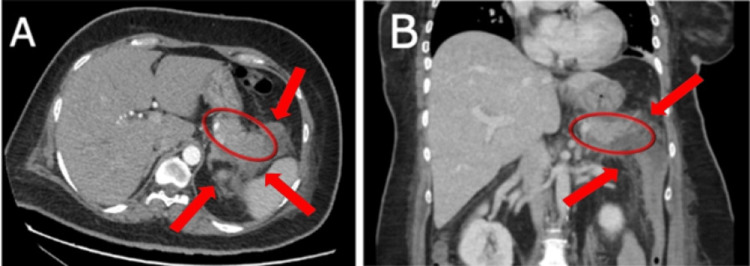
Contrast-enhanced computed tomography of the pancreas. A: Axial arterial-phase CT scan demonstrating a bulky pancreatic tail with ill-defined hypoenhancement on the arterial and portovenous phases (red oval), associated with peripancreatic fat stranding and free fluid (red arrows).
